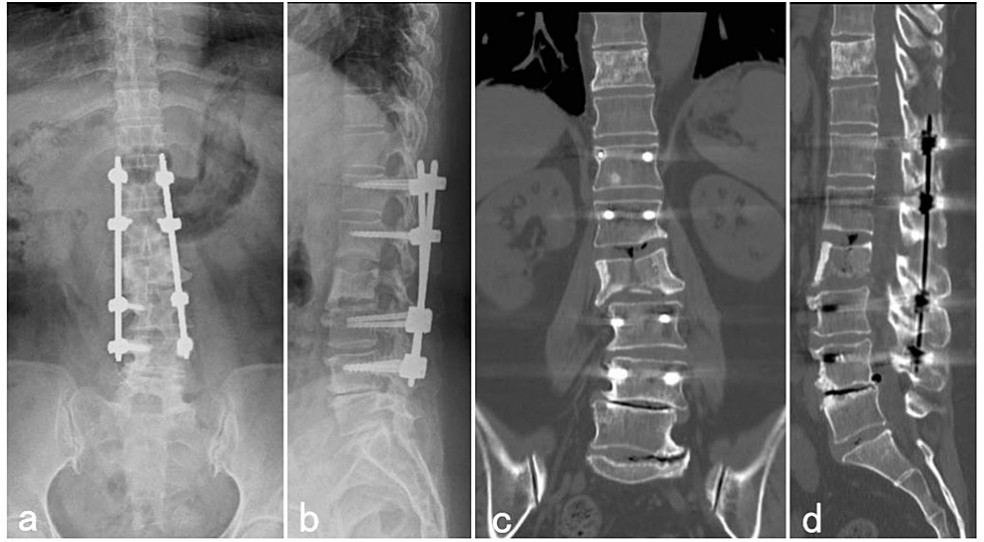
Plain radiograph and CT scan one year after surgery Postoperative anteroposterior (a) and lateral (b) radiographs show no loosening of the screws from L1 to L5 and osteosclerosis in T11 and L3 vertebral body. The coronal (c) and sagittal (d) CT imaging revealed complete osteosclerosis in the Th11 vertebral body and partial osteosclerosis and necrosis in the L3 vertebral body.
